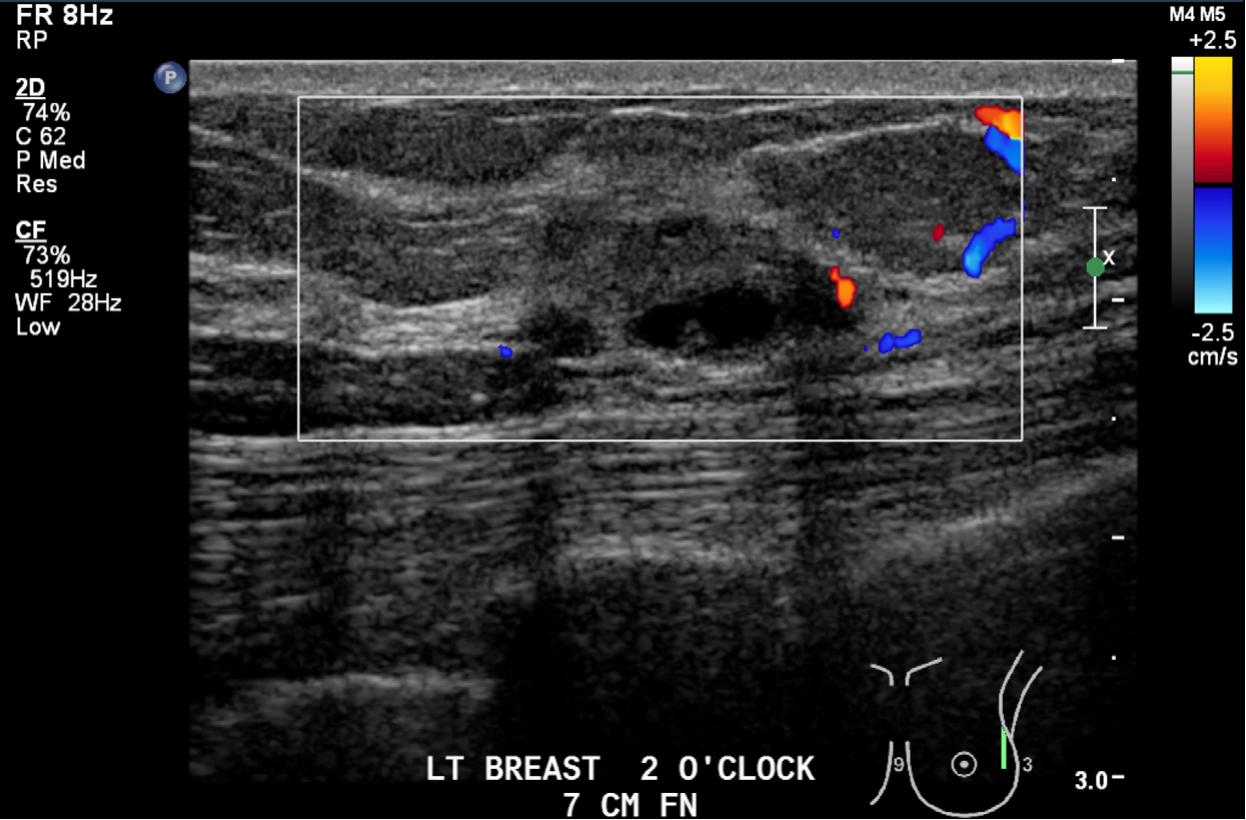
Lesion three with Doppler interrogation demonstrating avascular nature. Image courtesy of BreastScreen ACT.
